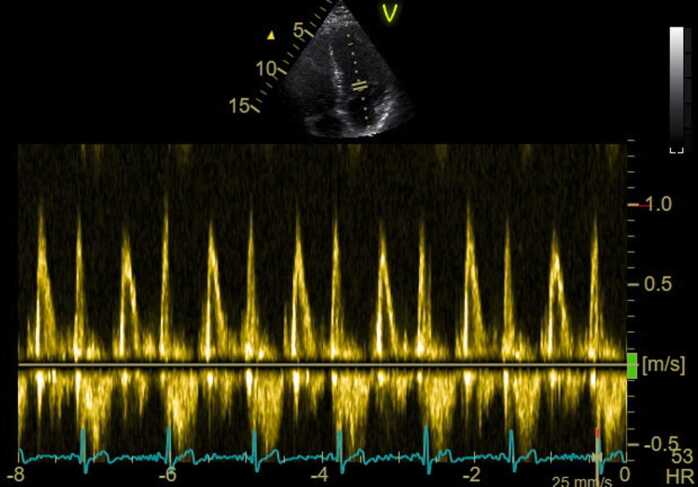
Example of E/A ratio assessment with echo, for the same patient as the one shown in Fig. 1. Parallel solid lines on the sector image indicate the manually placed location of the Doppler sample volume for velocity assessment at the level of the tips of the mitral valve leaflets. The heart rate during the examination was 53 beats per minute. HR, HeartRate.
